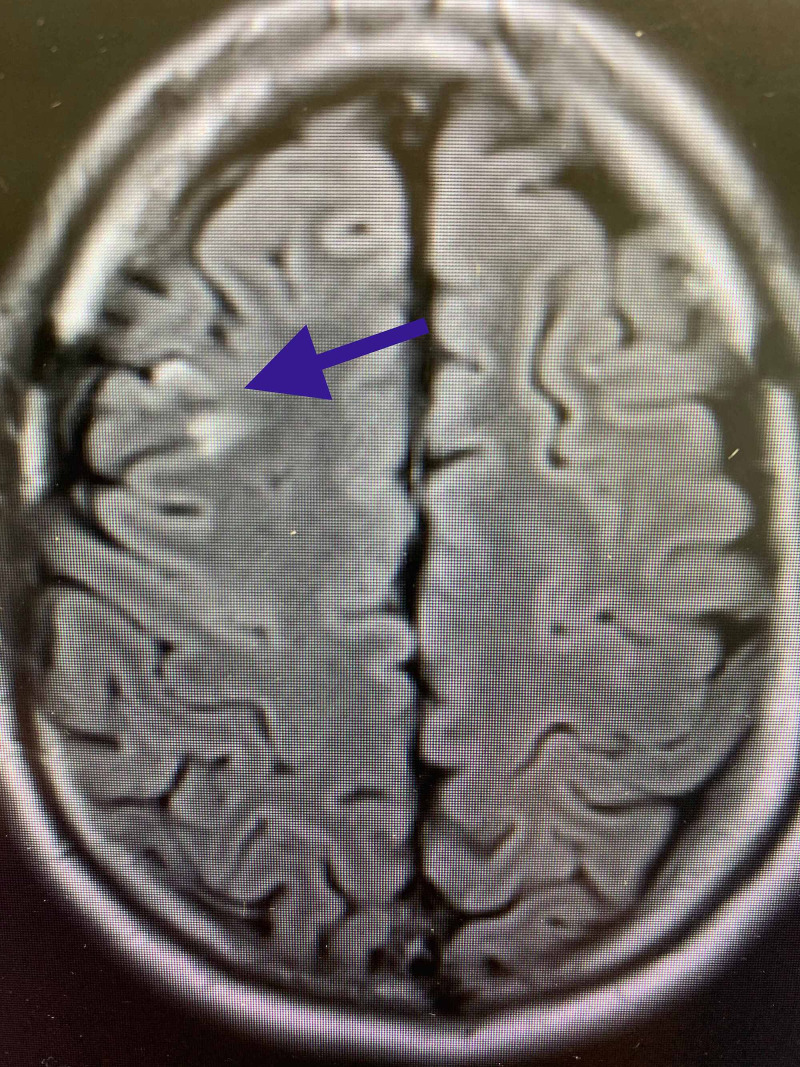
FLAIR MRI: right frontal cortical ischemic infarct (blue arrow). FLAIR MRI, fluid-attenuated inversion recovery magnetic resonance imaging
